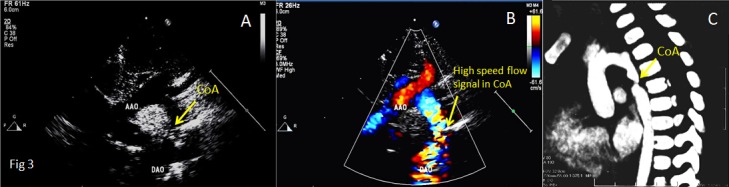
Transthoracic echocardiograms in suprasternal view of a 4-month-old boy showing [A] the location of the CoA between AAO and DAO and [B] the high speed flow signal in the CoA. Computed tomographic angiography [C] showed the location of the CoA. Abbreviations as in Fig 2.
